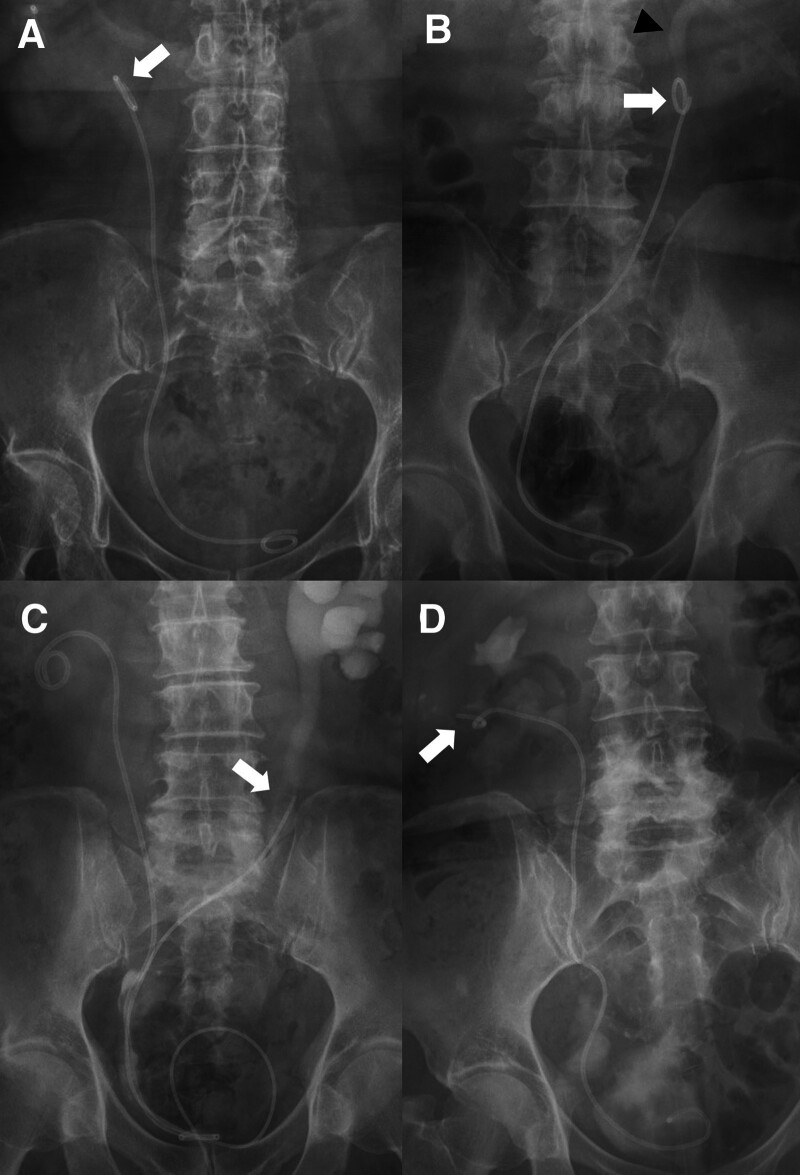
Double-J ureteral stent (DJUS) migration type observed abdomen X-ray. (A) Type I: the proximal tip (arrow) of DJUS located at the ureteropelvic junction. (B) Type II: the proximal tip (arrow) of DJUS located at the proximal ureter (arrowhead). (C) Type III: the proximal tip (arrow) of DJUS located at the mid to distal ureter. (D) Type IV: proximal migration of the proximal tip (arrow) of DJUS.
